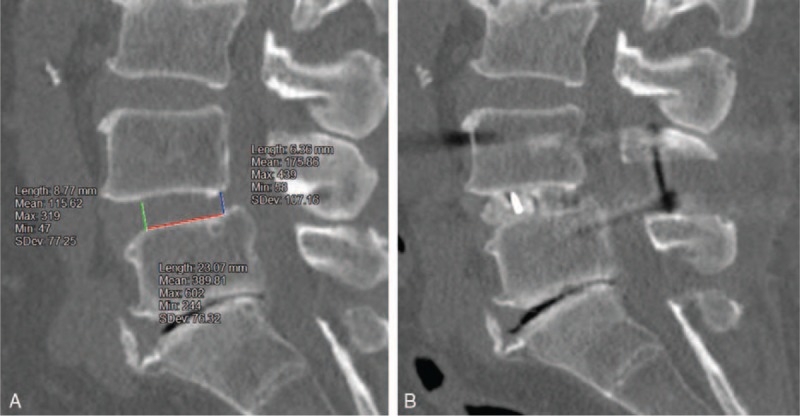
Example of selection of interbody cage height. (A) Measurement of anterior and posterior disc height on a preoperative CT scan. In this case, the anterior and posterior disc heights were 8.77 mm 6.36 mm, respectively. Thus, we selected cages with 8/6 mm (Height) × 9 mm (Width) × 21 mm (Length). (B): Postoperative CT scan. In this case, the L4-L5 vertebral height remained unchanged perioperatively (minimum disc distraction). CT = computed tomography.
